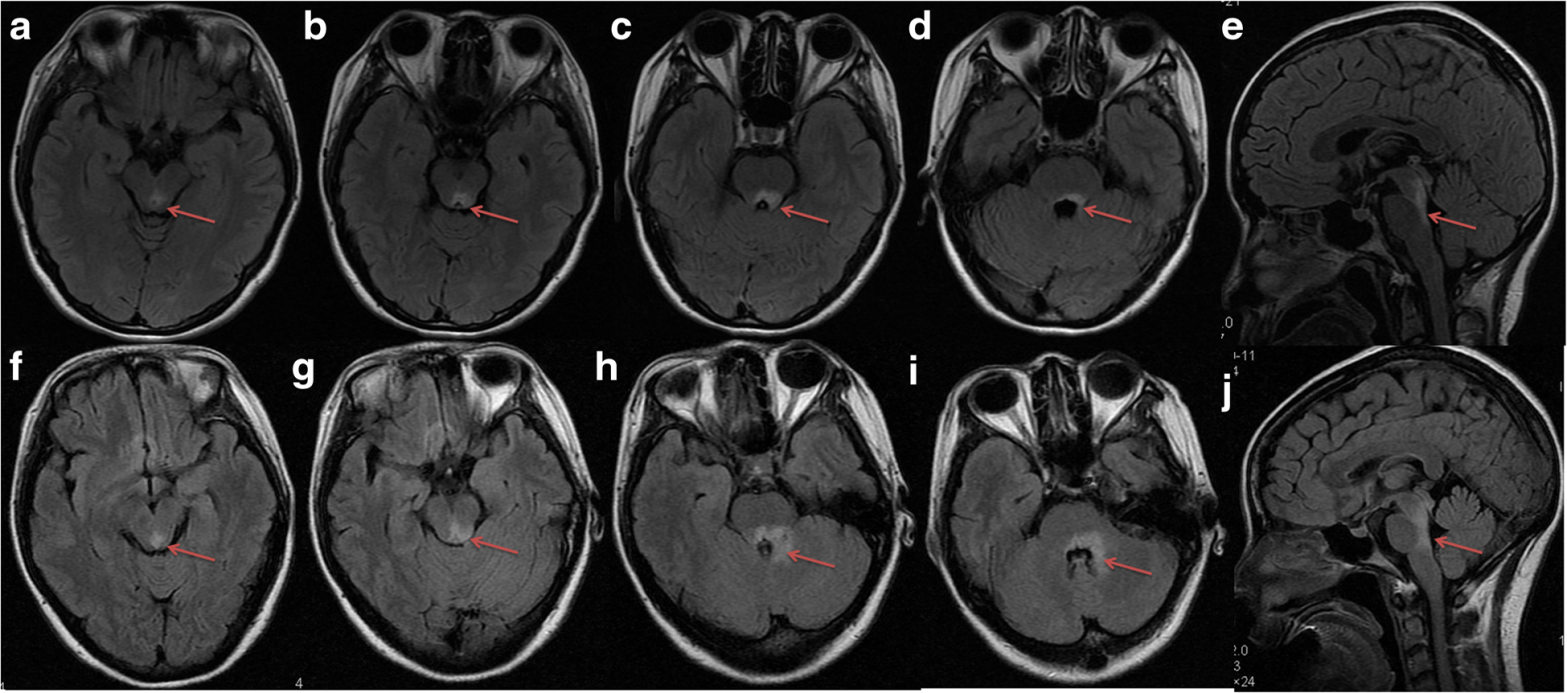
Axial T2-weighted FLAIR MRI shows periependymal lesions are involved in the dorsal midbrain (a, b and f, g; *arrow*) and pons (c, d and h, i; *arrows*). Sagittal T2-weighted FLAIR MRI shows increased signal surrounds the fourth ventricle (e and j; *arrows*)
